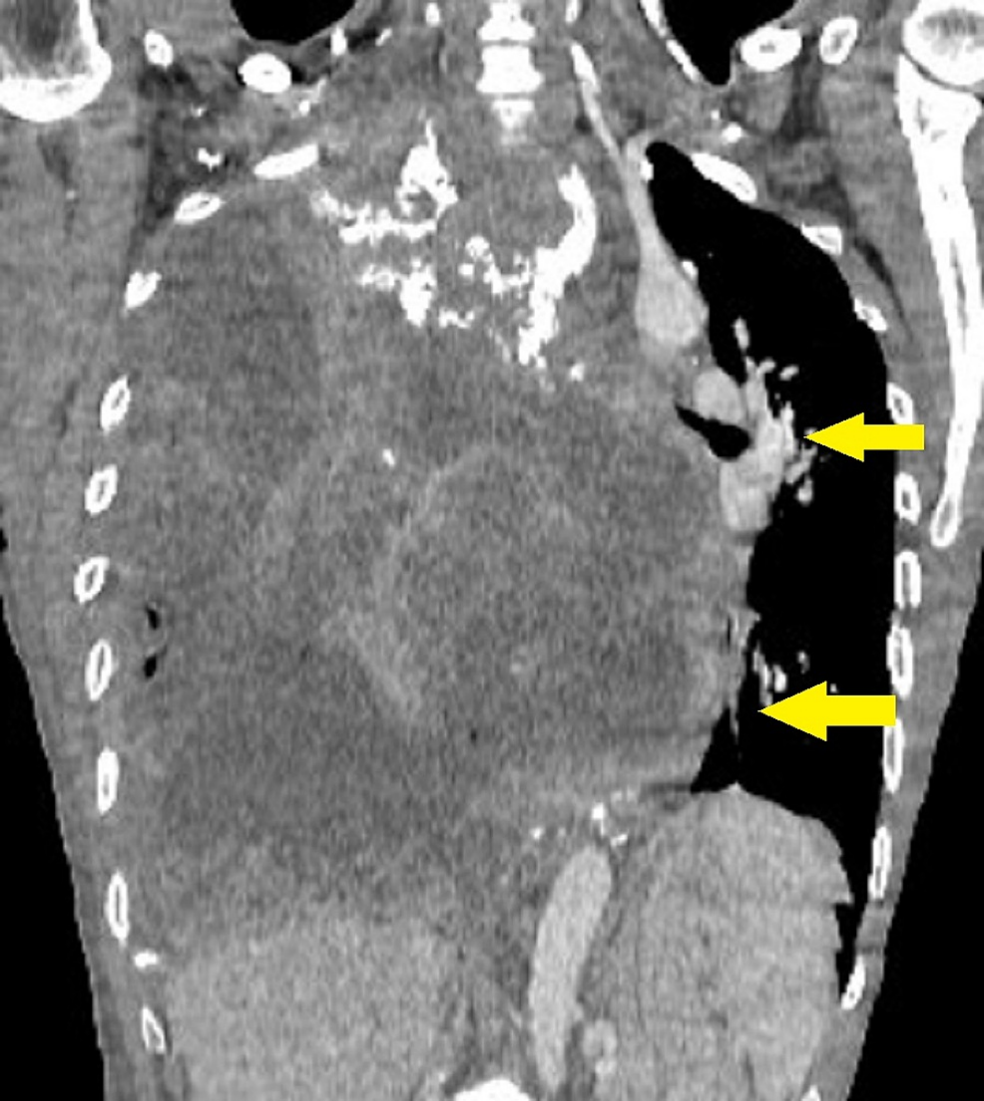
A coronal plane of the CT scan with injection of intravenous contrast showing the tumor occupating all the right hemithorax (arrows showing the deviation of the mediastinum).
